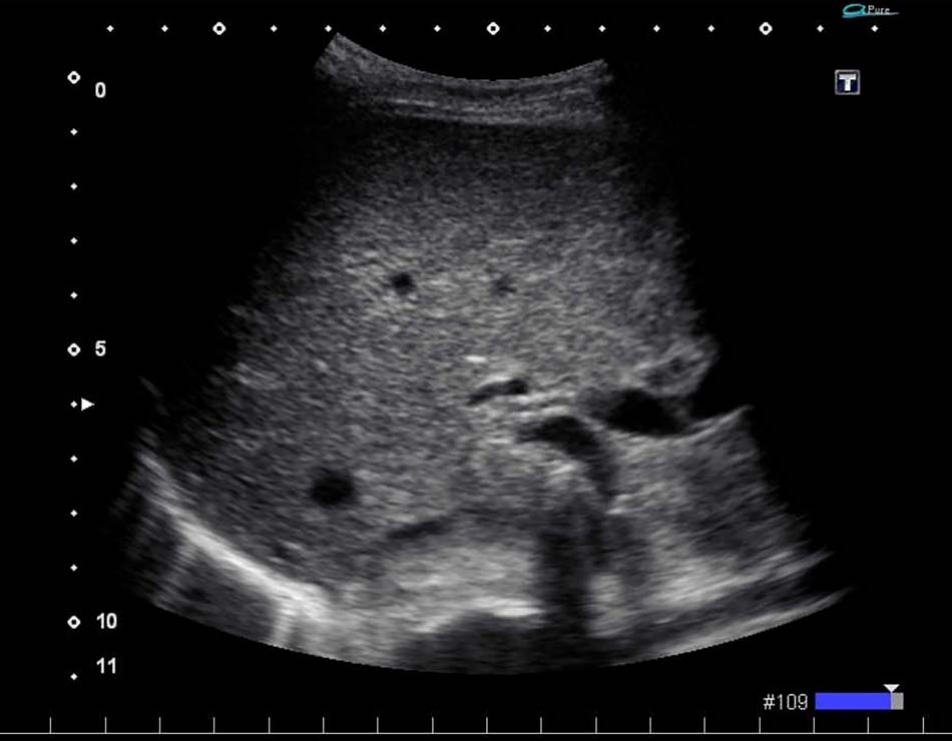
Ultrasonography after surgery shows no findings of PVG in the liver.
